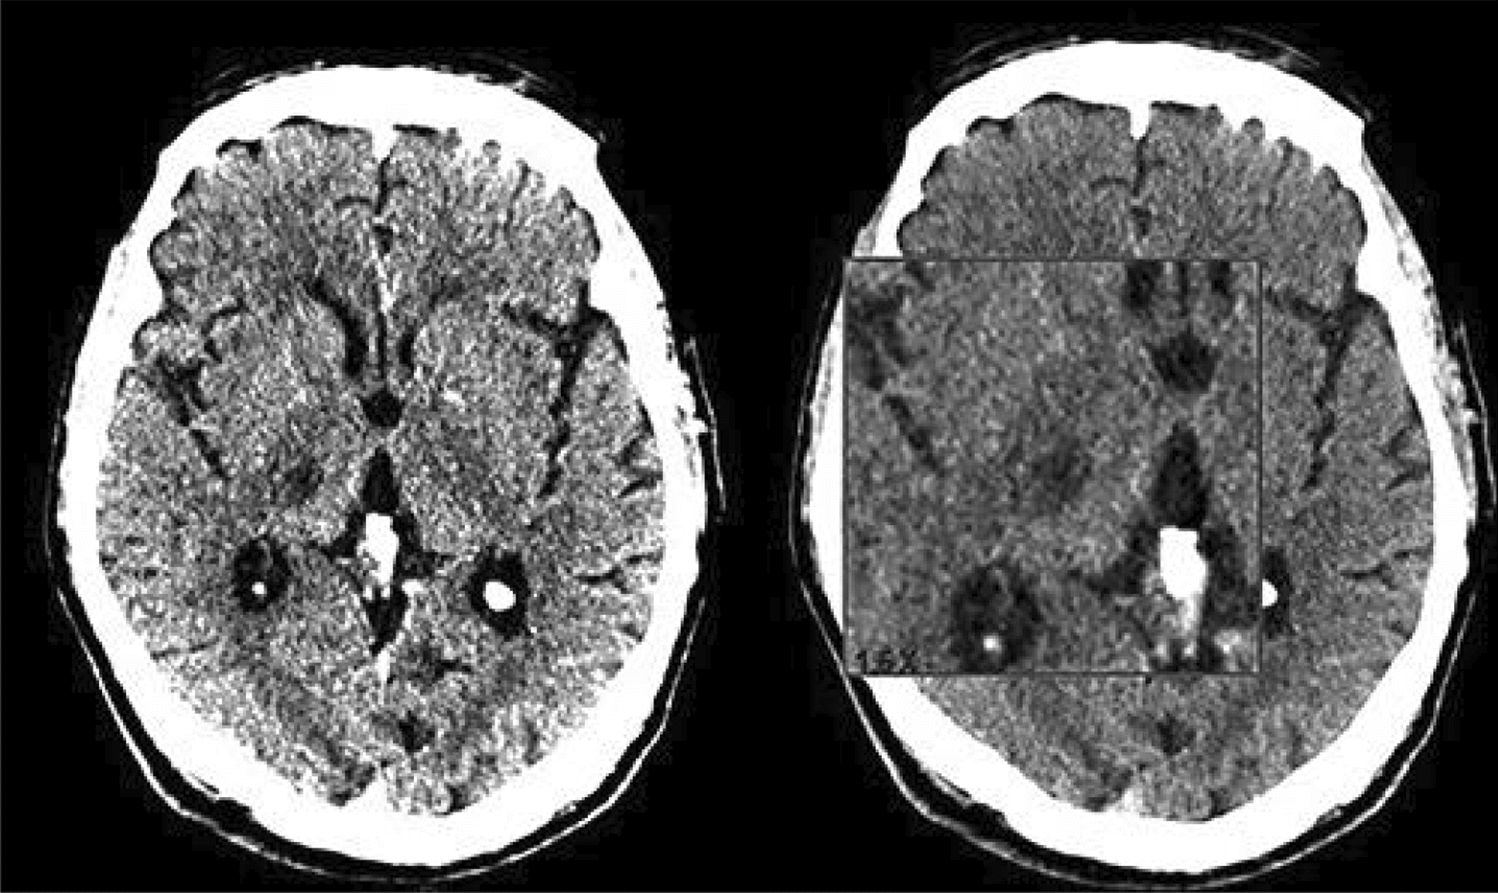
24-h computed tomography brain scan showing a new-onset right thalamic ischemic lesion [55]
